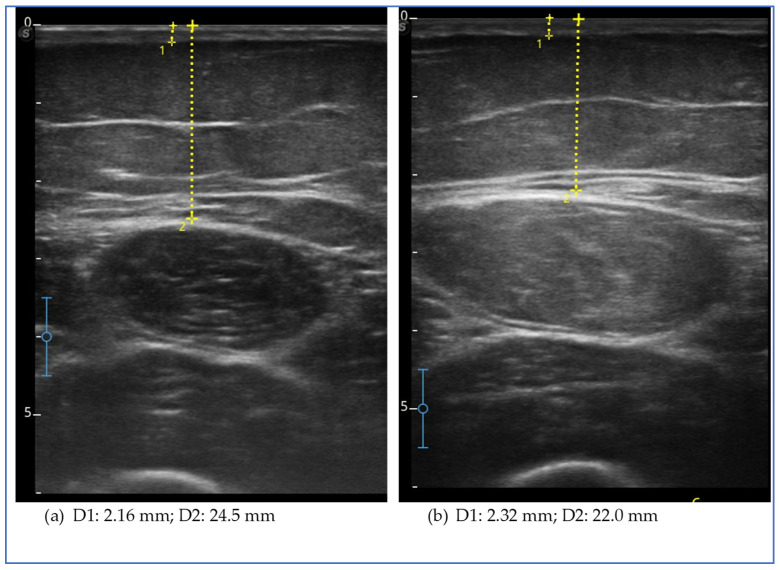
Ultrasound measurements for case report 1.
